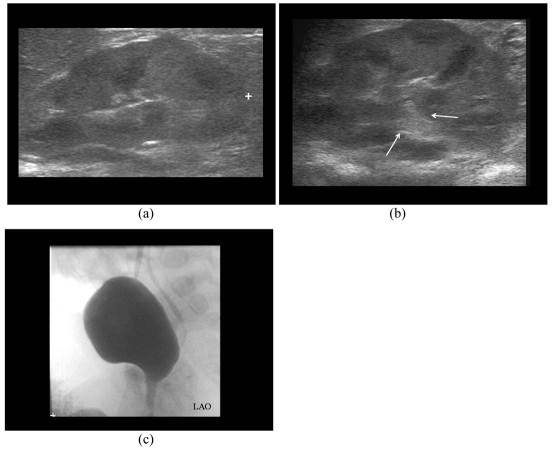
A four months-old girl who had E. coli urinary tract infection. a) Right sonogram shows the PCS is not dilated; b) ECS shows presence of microbubbles in dilated right PCS (arrows); c) MCU demonstrates bilateral Grade II VUR (arrows). The left Grade II VUR was seen as presence of microbubbles in non-dilated PCS on ECS.
